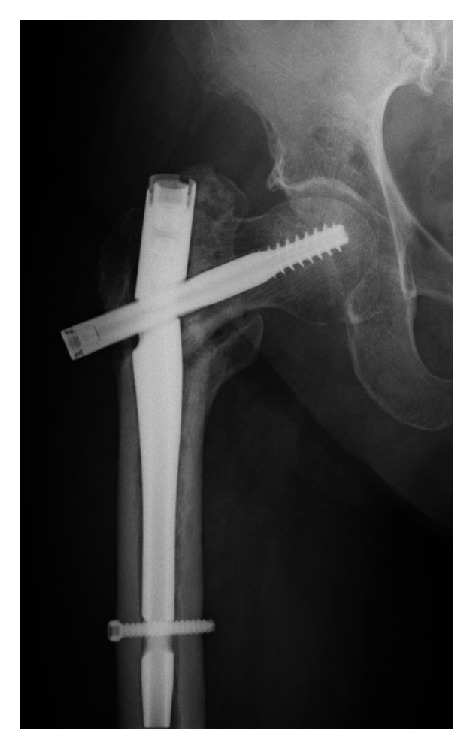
Radiograph showing nail breakage at the opening for the lag screw at 14 months after surgery. The fracture shows signs of nonunion with sclerosis of the bone ends.
